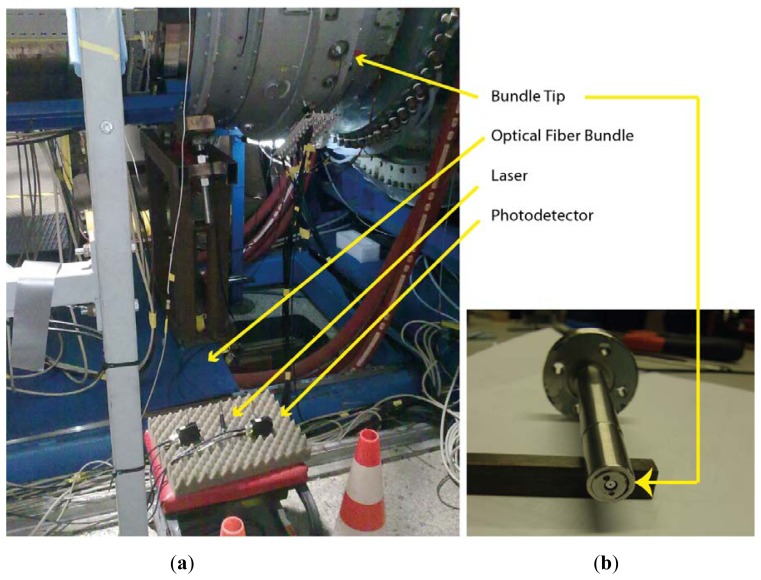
(a) Experimental setup for the tip clearance and tip timing measurements in the turbine rig at the CTA facilities. (b) Coupler to fix the bundle tip to the turbine casing.
